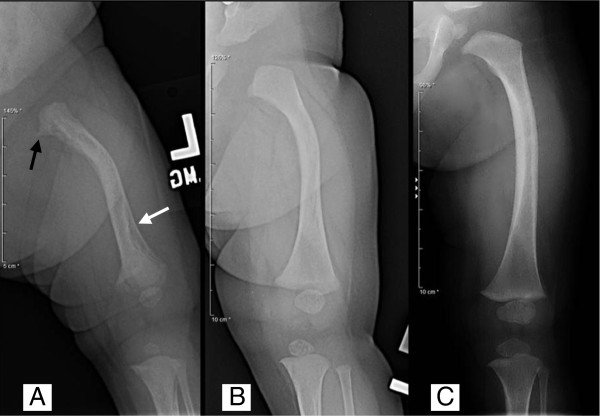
Changes in radiographic findings of the left femur over the course of observation (age 2 weeks to 1 year). A. 2 weeks: antero-lateral bowing, widened and irregular metaphyses with flaring and medial beaking (black arrow), and thick, smooth periosteal reaction (white arrow). B. 3 months: improved bowing, resolved periosteal reaction and mild residual beaking of the medial upper femoral metaphysis. C. 1 year: Further improvement in lateral bowing, complete resolution of metaphyseal deformity.
